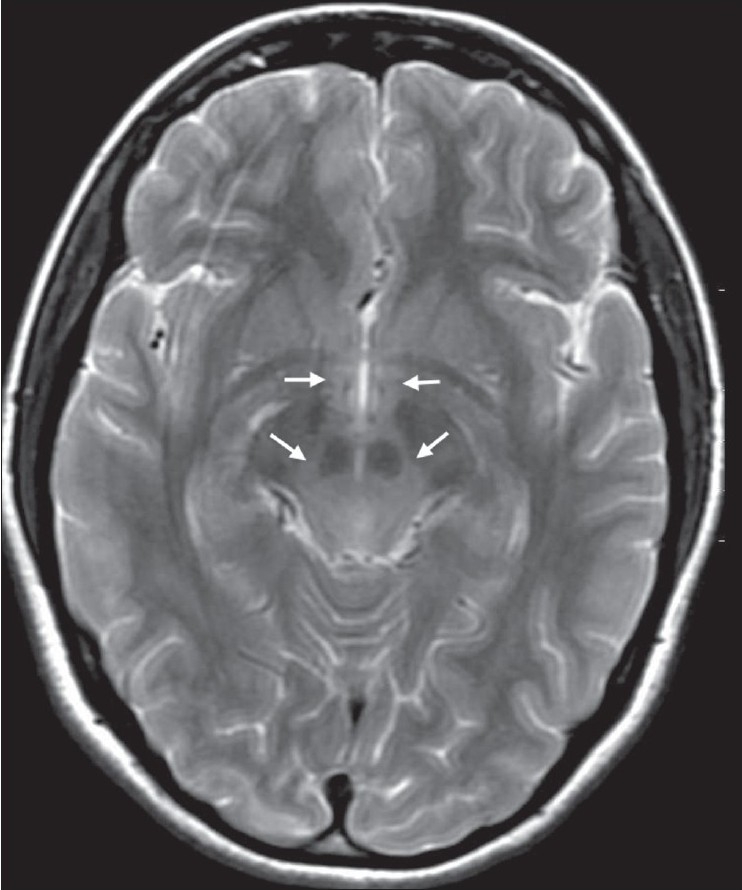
Axial T2W MRI image reveals hyperintensity in the midbrain and hypothalamus (arrows)
